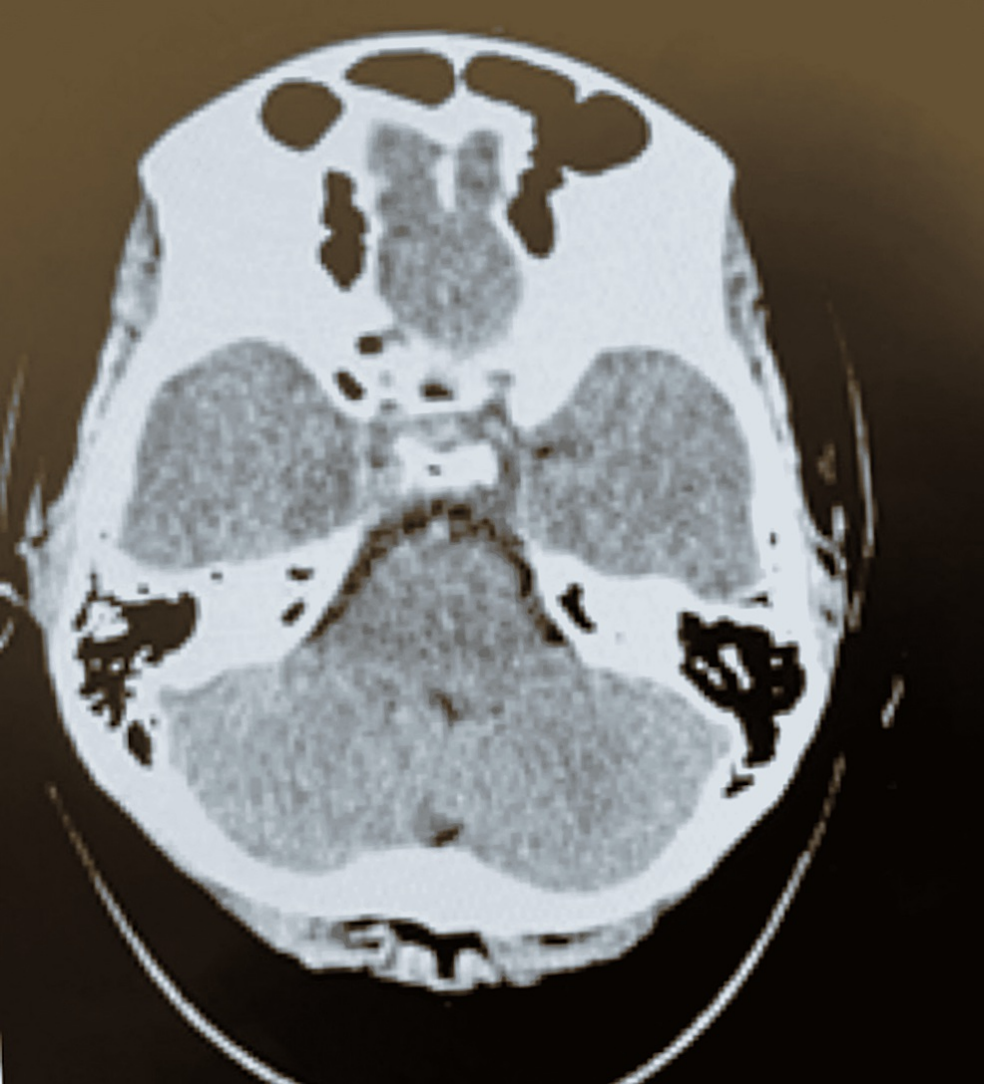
CT of the brain without contrast The axial view shows normal gray and white matter in the brain parenchyma with no evidence of cerebral injury, infarct, or intracranial hemorrhage.
